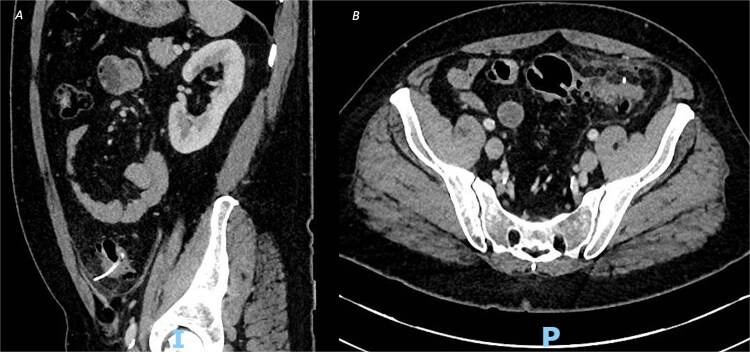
CT of the abdomen and pelvic with contrast, demonstrating an ingested foreign body with localized perforation and pericolic inflammatory changes, in both (A) sagittal view and (B) axial view.
